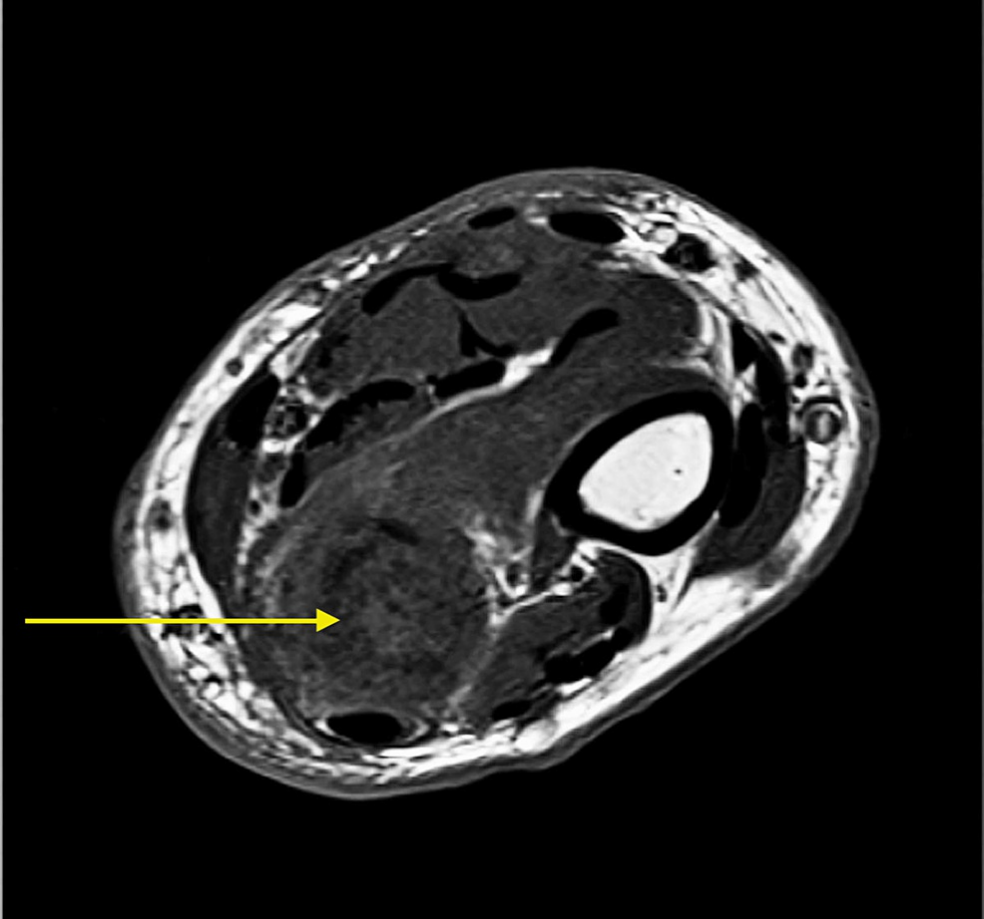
Pre-operative T1 weighted MRI of the right forearm in axial view. Shows complete displacement of marrow fat with tumor and severe cortical thinning.
